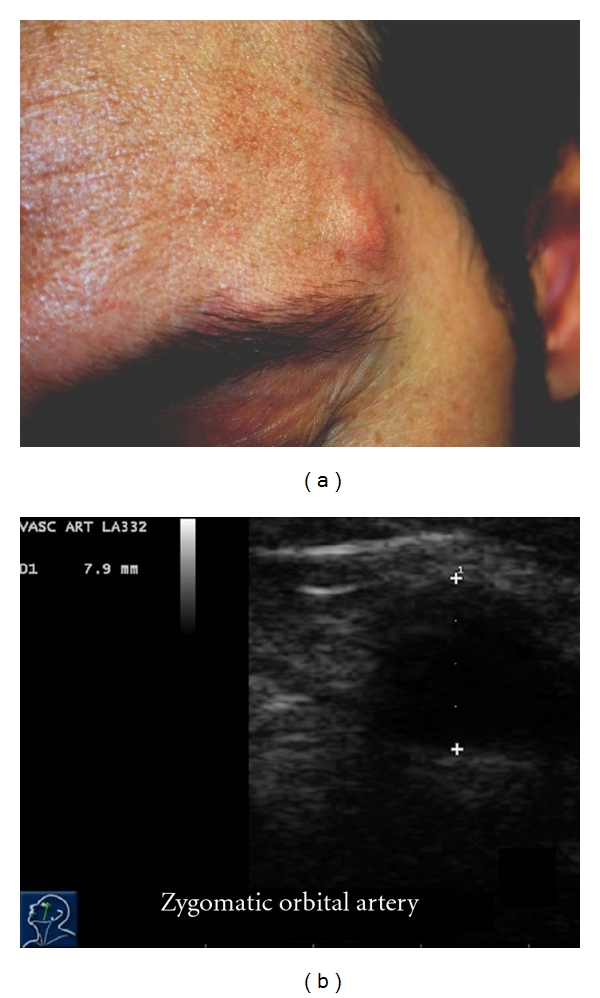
(a) Pulsatile subcutaneous mass on the upper lateral border of the left orbital arch. (b) Cross section of zygomatic orbital artery aneurysm at preoperative echo color Doppler.
